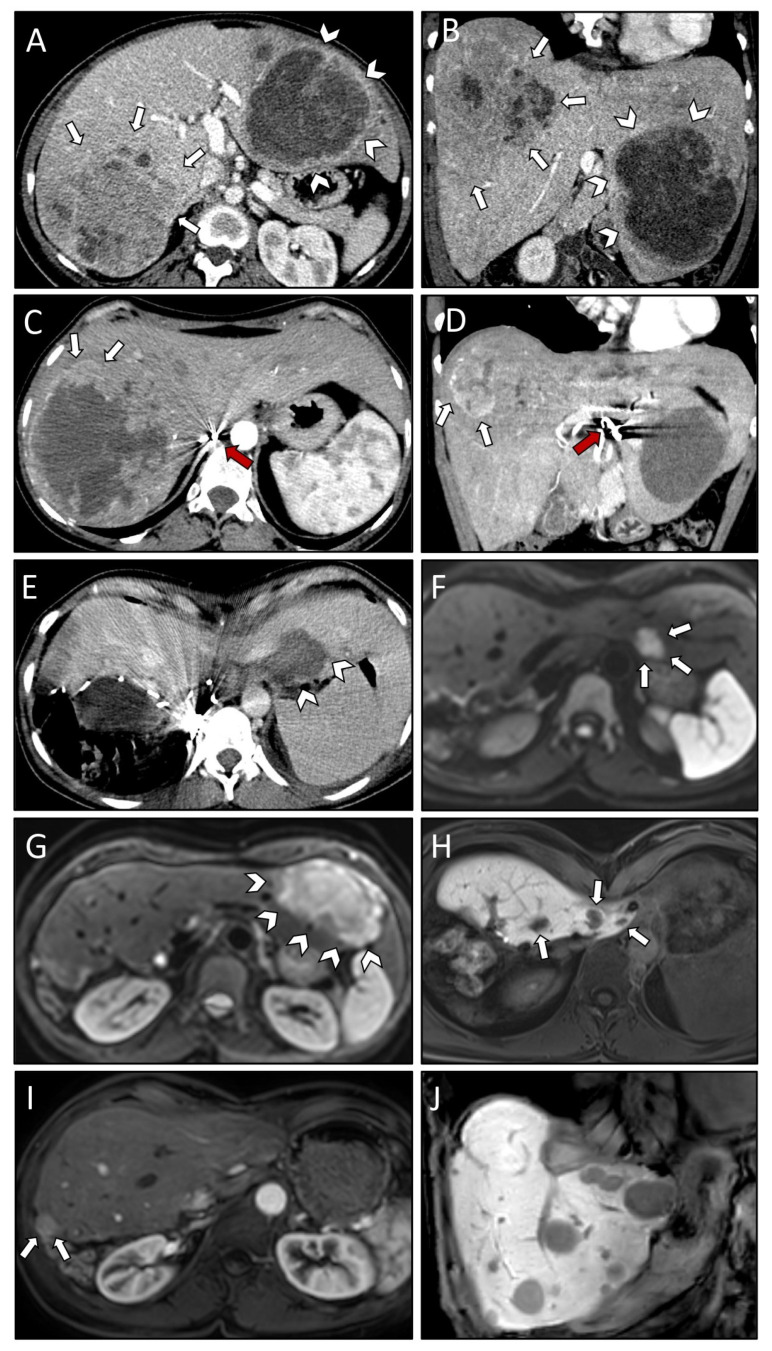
Axial (A) and coronal plane (B) of initial CT scans demonstrating very large SFT/HPC liver metastases in both right (white arrows) and left (white arrowheads) liver lobe. CT scan in axial (C) and coronal plane (D) after three TAE sessions revealing insufficient devascularization of the right liver lobe lesion anteriorly (white arrows). Note the beam hardening artifacts (red arrows) in the hepatic artery. Post-interventional CT scan (E) after first SRFA with laparoscopic liver packing (protection of cardio-esophageal region) showing coagulation zone (white arrowheads) with no residual vital tumor tissue visible. Contrast-enhanced MRI scan four months after first SRFA revealing a new metastasis in liver segment II (white arrows; (F)) and local recurrence in segment III following TAE (white arrowheads; (G)). MRI scan depicting three of six new liver lesions in segment IVa (white arrows; (H)) after resection of left lateral segments, all of them successfully treated with SRFA. MRI scans 15 months after the last intervention showing local recurrence at the resection margin (white arrows; (I)) and multiple new liver metastases (J).
